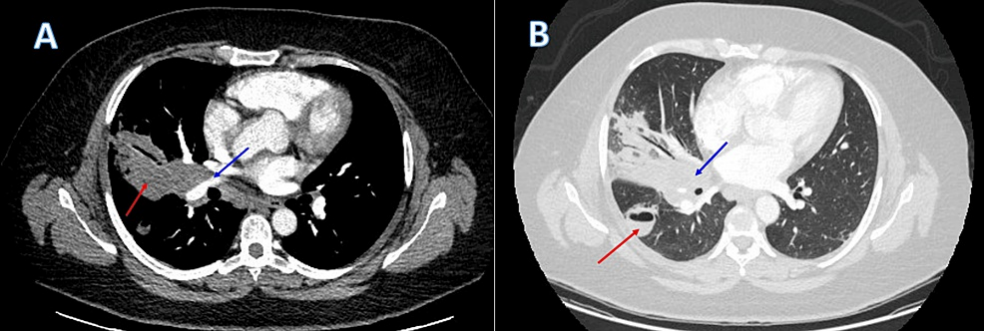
CT scans following bronchoscopy (A) Soft tissue window showing RML consolidation with air bronchogram (red arrow). The proximal anterior RLL pulmonary artery is encased within the right hilar mass and mildly compressed; (B) Lung window showing RLL cavitary lesion with air fluid level (red arrow) and RML consolidation with air bronchogram (blue arrow).
